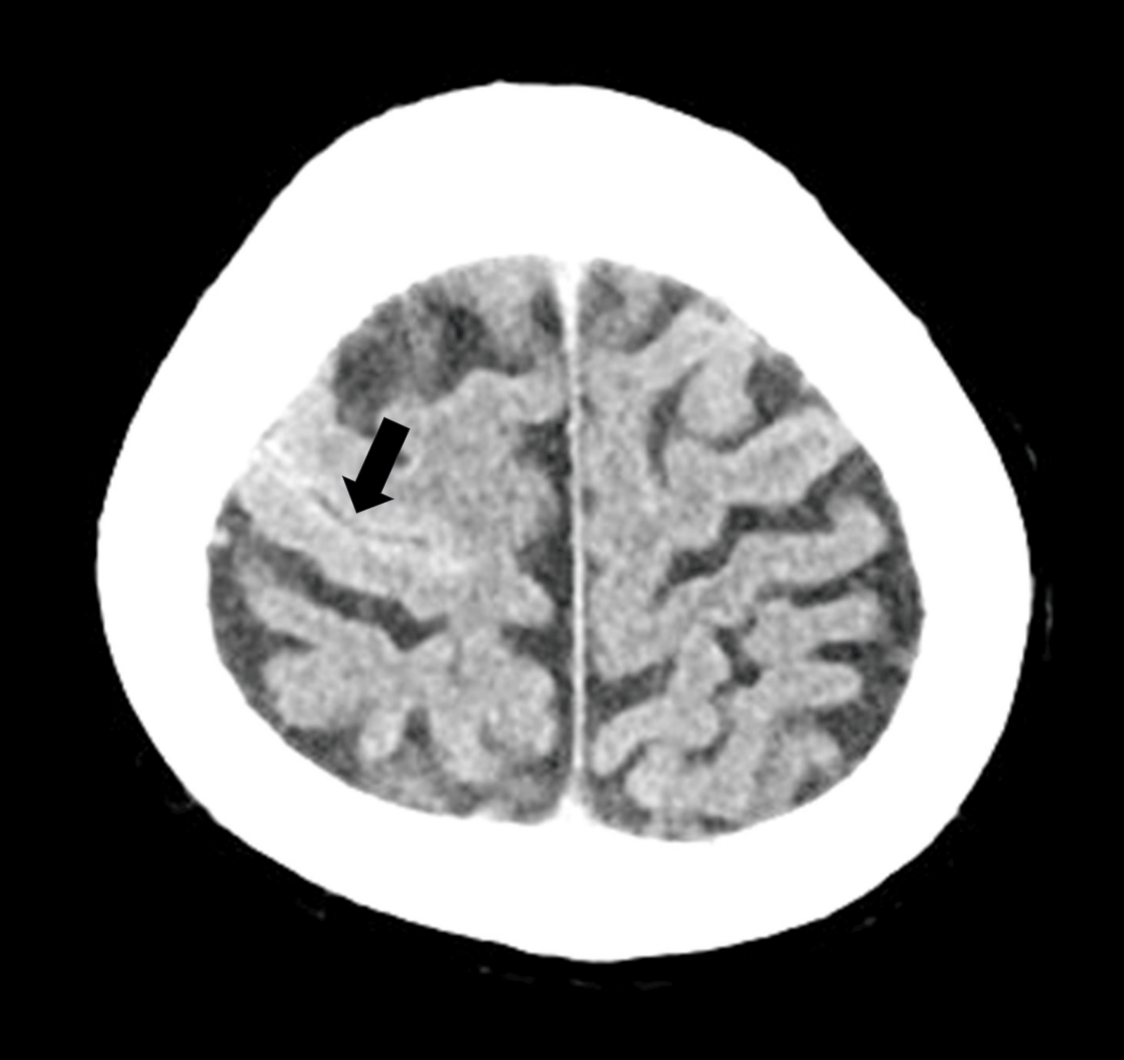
Head CT (Case 1). Non-contrast-enhanced axial head CT on admission showing localized subarachnoid hemorrhage centered around the right precentral sulcus (black arrow).
